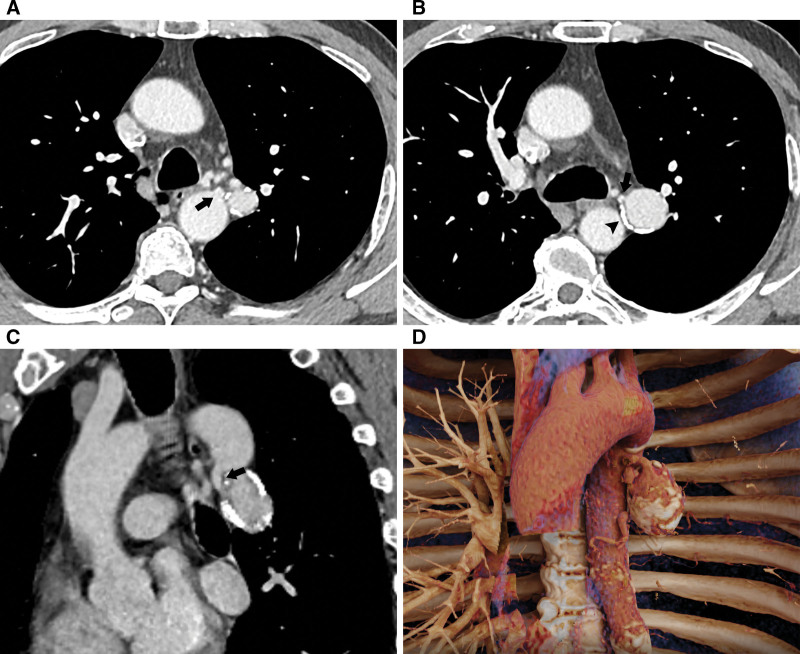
(A) A single enlarged bronchial artery aneurysm (BAA) has been identified in the left hilar region, originating from the thoracic aorta (black arrow). (B) Chest CT angiography (CTA) showed the BAA was surrounded by annular calcification (black arrowhead). Additionally, the neck of the aneurysm, adjacent to the aneurysmal sac, appeared relatively narrow (black arrow). (C) The oblique coronal chest CTA (left anterior oblique angle of 30°) distinctly revealed a short, funnel-shaped dilated aneurysm neck. (D) The 3D-VR image clearly showed the location and size the detected BAA. BAA = bronchial artery aneurysm, CTA = computed tomography angiography, VR = three-dimensional virtual reality.
